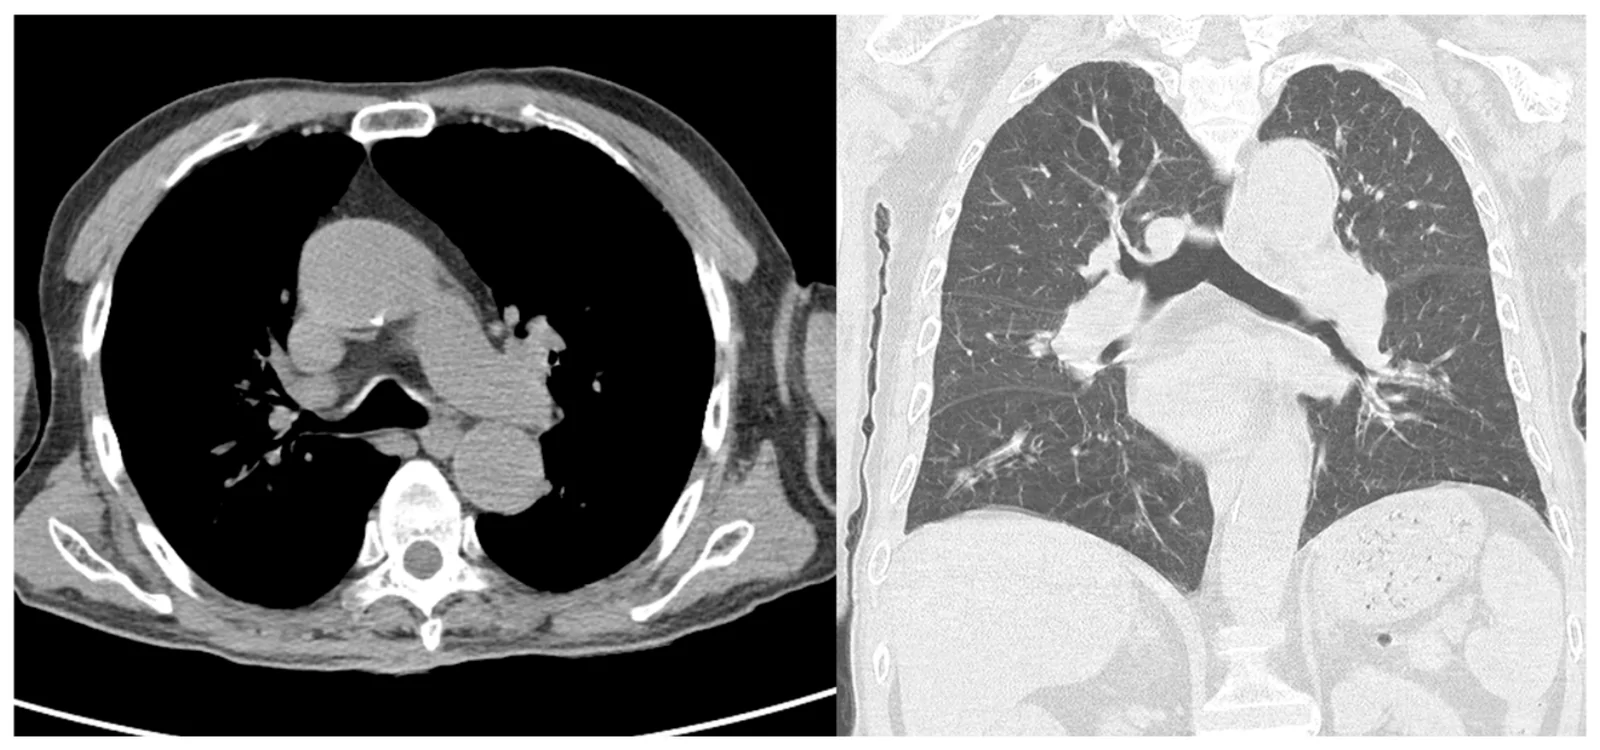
Follow-up chest computed tomography demonstrating disappearance of the previously identified foreign body in the right main bronchus after routine endotracheal suctioning on axial and coronal CT images. No obvious associated pulmonary abnormalities, including pneumothorax or atelectasis, were identified.
